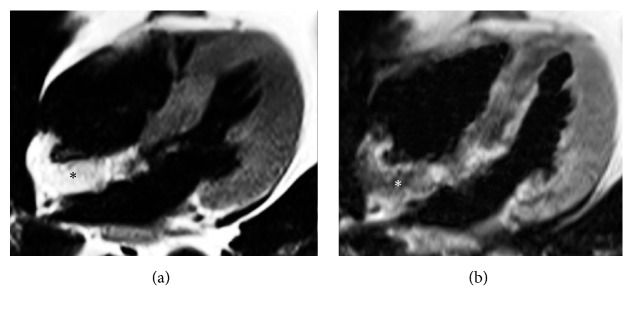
CMR exam in a lipomatous hypertrophy of the interatrial septum in an asymptomatic 68-year-old female. Four-chamber black blood T1-weighted image (a) shows a hyperintense lobular mass infiltrating the interatrial septum and part of the posterior wall of the right atrium (asterisk). Corresponding T1-weighted image with fat suppression (b) demonstrates homogeneous signal drop of the mass, confirming the presence of adipose tissue (asterisk).
